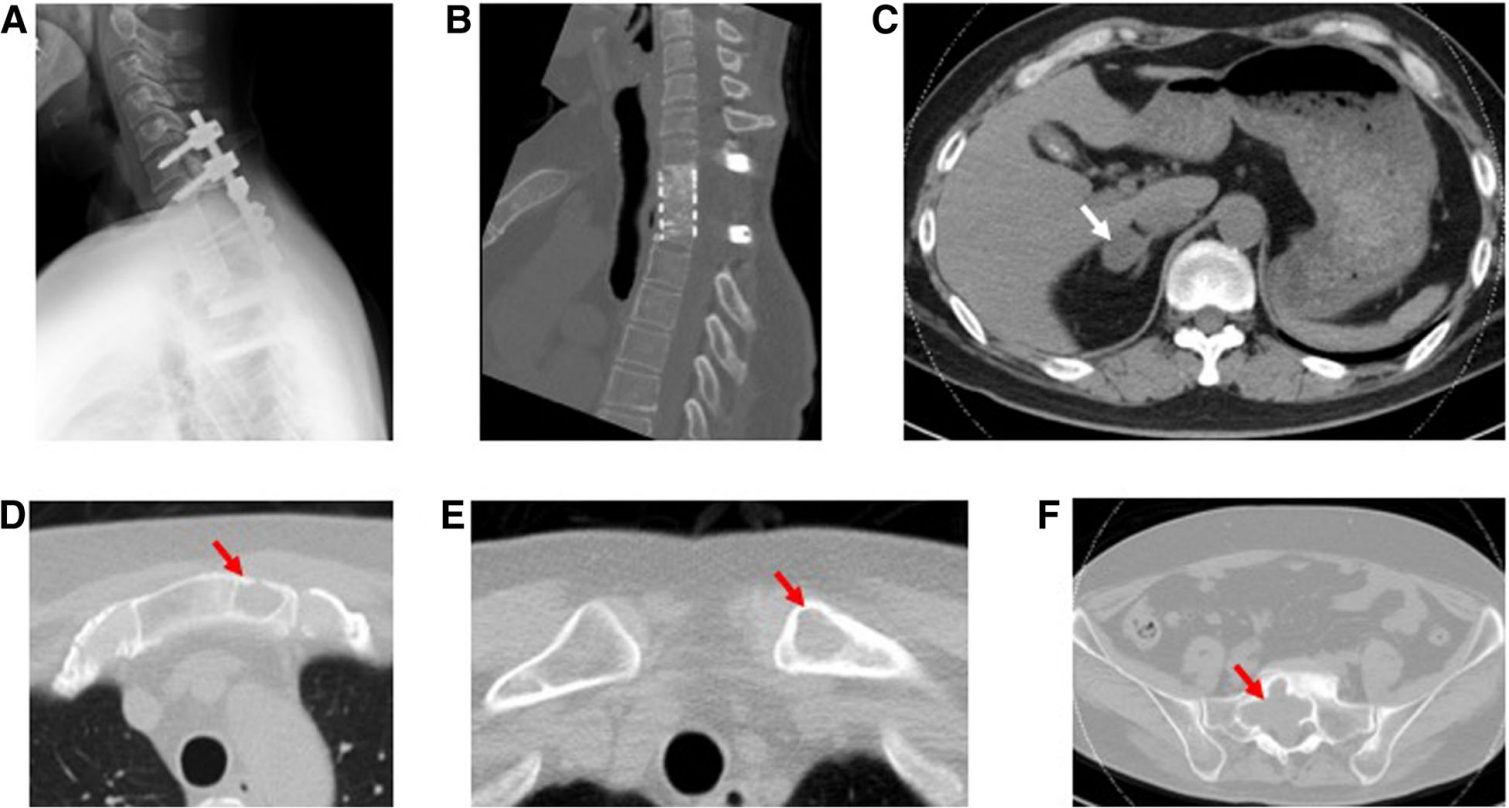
Postoperative imaging at follow-up, 5.5 years after total *en bloc* spondylectomy, showing well-maintained instrumentation (a) with radiographic bony union between adjacent vertebrae and the bone graft (b). There is no evidence of local recurrence. Axial computed tomographic images demonstrate the increasing size of the right adrenal gland metastasis (c) but stable metastases in the sternum (d), left clavicle (e), and sacrum (f) (*arrows*)
